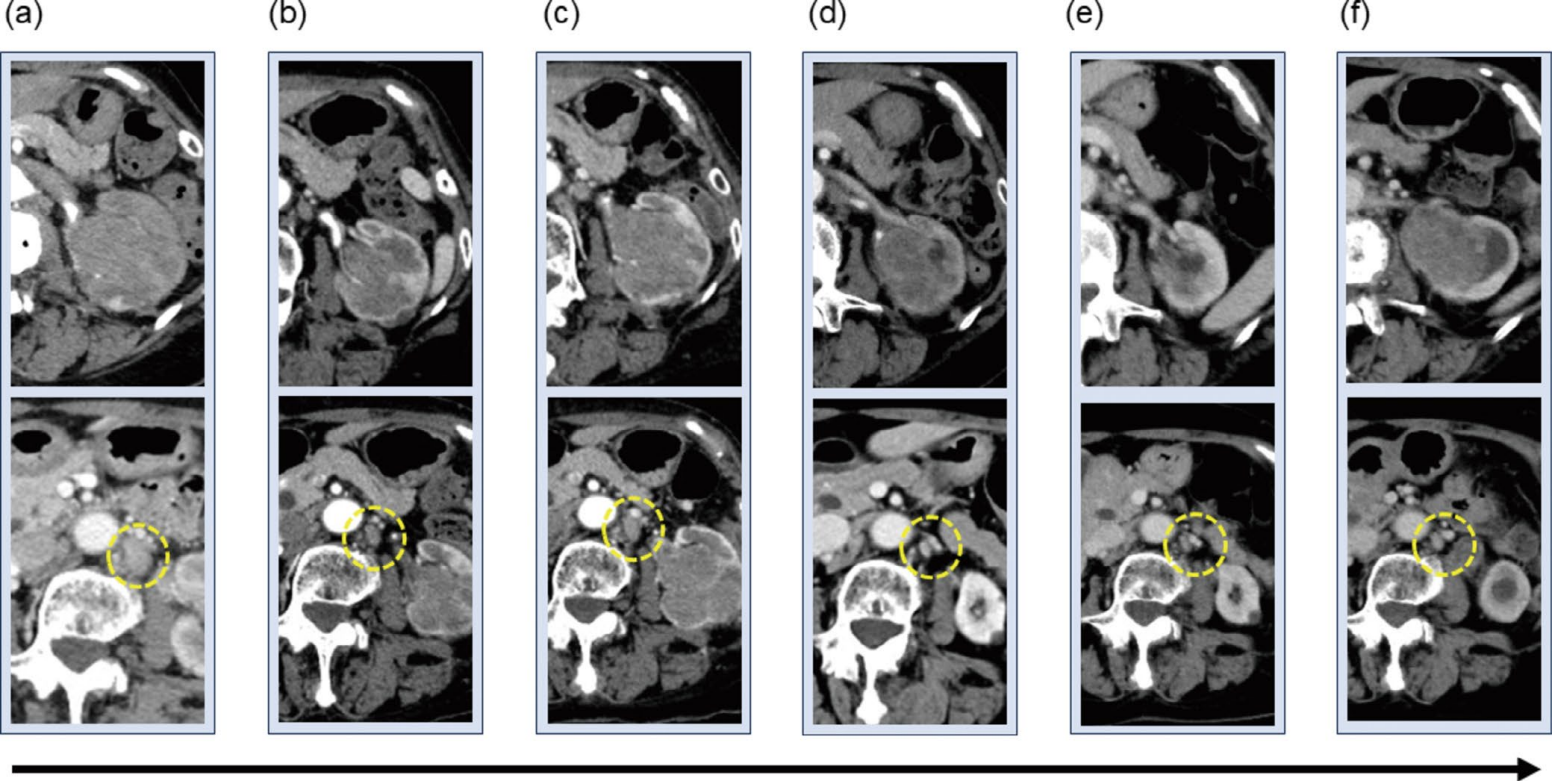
(a) CT image at diagnosis. (b) CT image after two cycles of gemcitabine and cisplatin. (c) CT image after five cycles of gemcitabine and cisplatin. (d) CT image after 11 cycles of pembrolizumab. (e) CT images after three cycles of EV following achievement of PR. (f) CT image before robot‐assisted nephroureterectomy.
